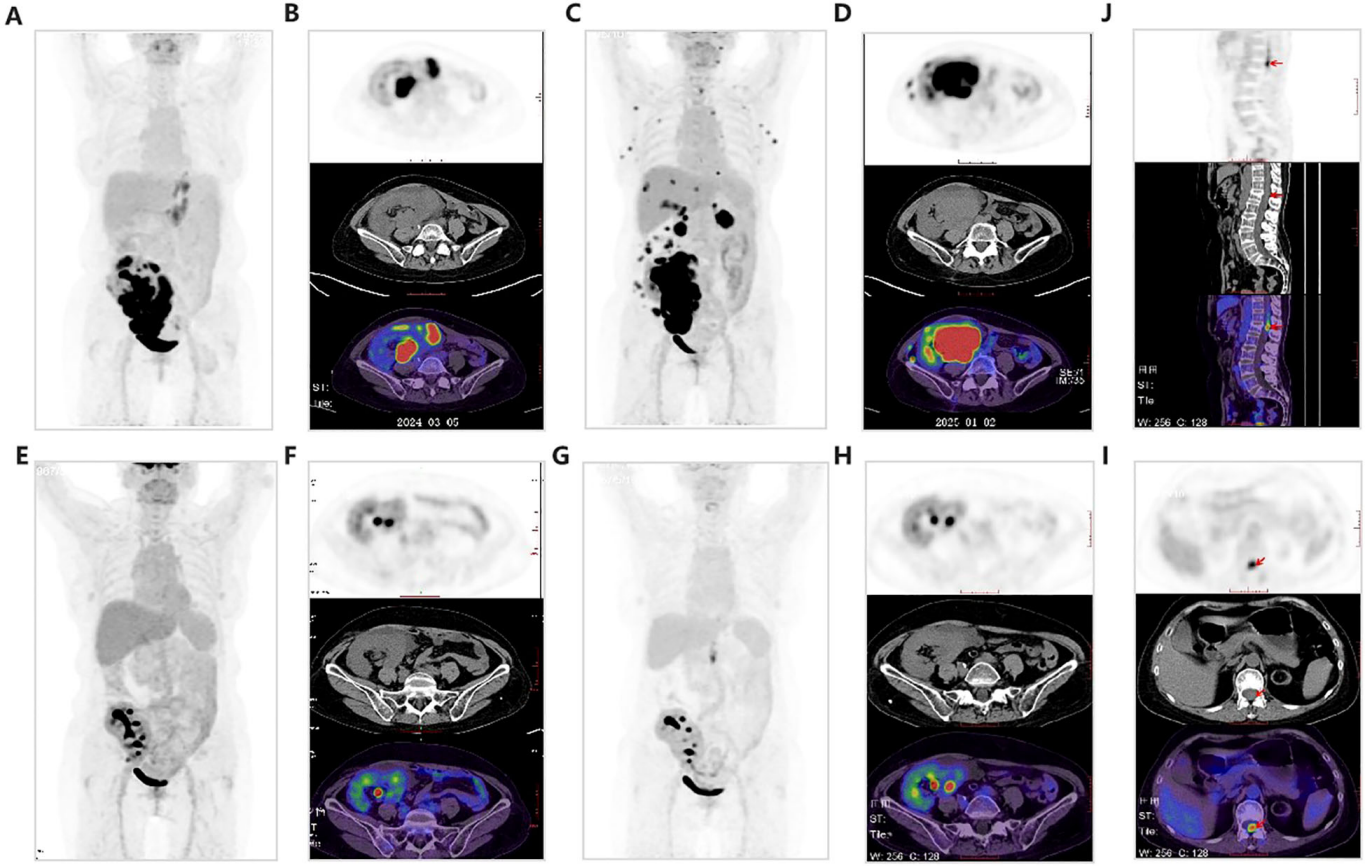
Nuclear medicine imaging. (A, B) PET/CT at initial diagnosis. (C, D) PET/CT at disease progression, 10 months after diagnosis. (E, F) PET/CT after three cycles of Pola-R-CHP, 13 months after diagnosis. (G–J) PET/CT at 17 months after diagnosis, two months after completion of six cycles of chemotherapy.
